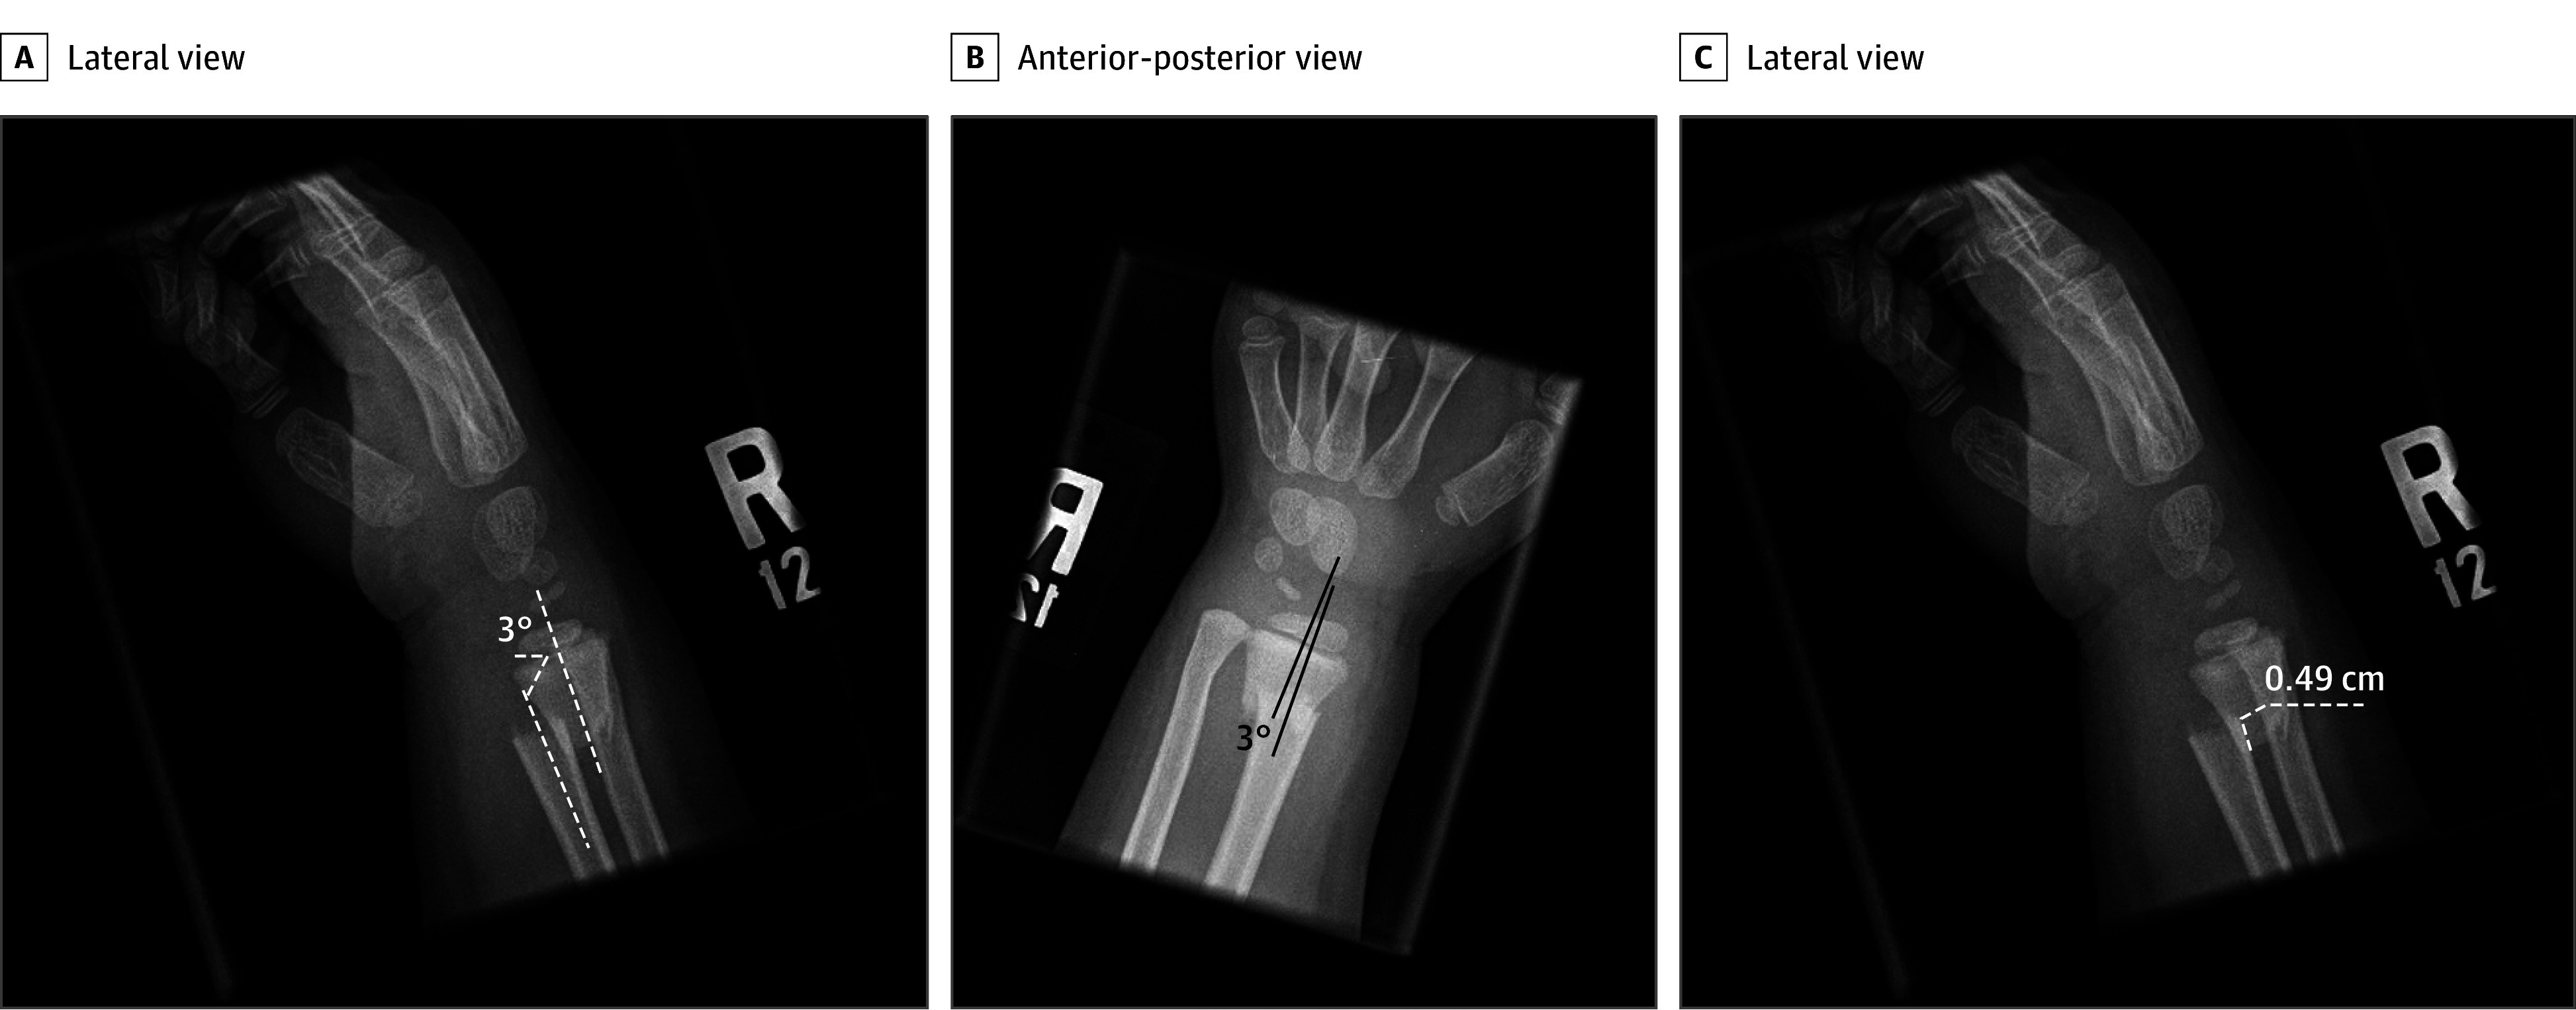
Radiographs of a Potentially Unnecessary Reduction
